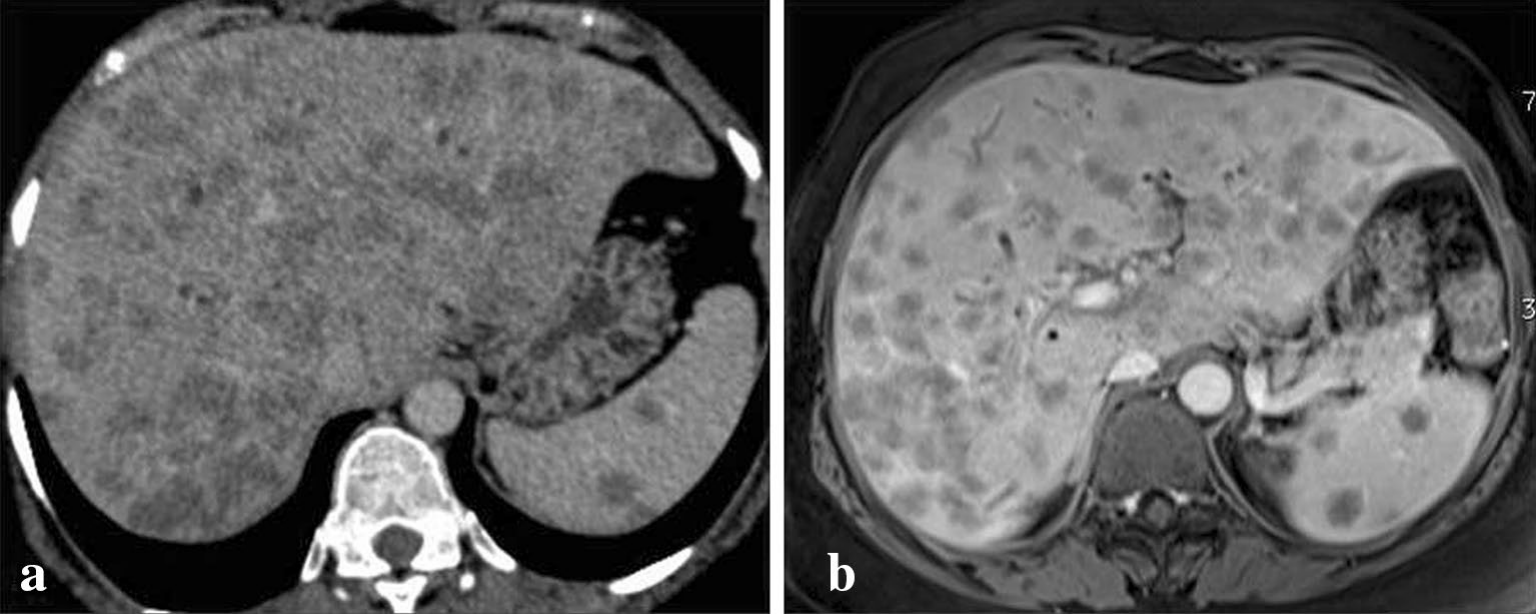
Multiple hepatic and splenic nodules hypodenses in the scanner (a) and hypointense on MRI with a delayed enhancement (b)
